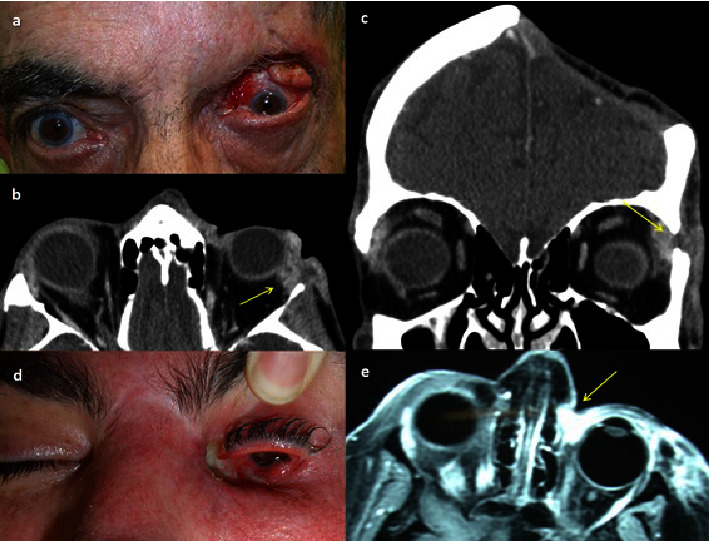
(a) Recurrent basal cell carcinoma (BCC) in upper eyelid treated with radiotherapy (RT) (patient 4). Ulcerated mass that has eroded upper eyelid and periocular tissue up to the orbital roof, adherent to deep planes, and with downward displacement of the eyeball. (b) Axial computed tomography (CT) scan demonstrates a soft tissue mass in the left outer canthus of the left orbit. The lesion shows mild enhancement with irregular borders. Skin is infiltrated at the level of the superior eyelid with lacrimal gland invasion (arrow). (c) There is bone destruction of the lateral margin of the roof (arrow) (patient 4). There is no fatty plane of separation with the sclera and the anterior portion of the lateral rectus muscle. (d) Recurrent BCC with large ulceration in the medial canthus and part of the lower eyelid (patient 1). Limitation of ocular motility. (e) CT scan showing infiltration of the anterior orbit, lacrimal and ethmoid bones, sclera, and anterior portion of the medial rectus muscle (arrow).
